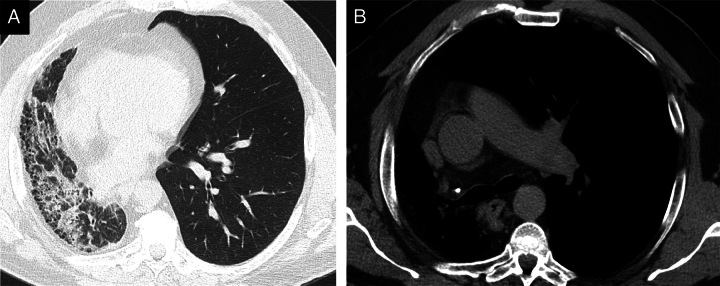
A 65-year-old man with right pulmonary artery agenesis. A, High-resolution CT shows extensive fibrotic changes and volume loss in the right lung, quantified to be 91.3% at DTA. B, The mediastinal window shows the absence of the right pulmonary artery.
